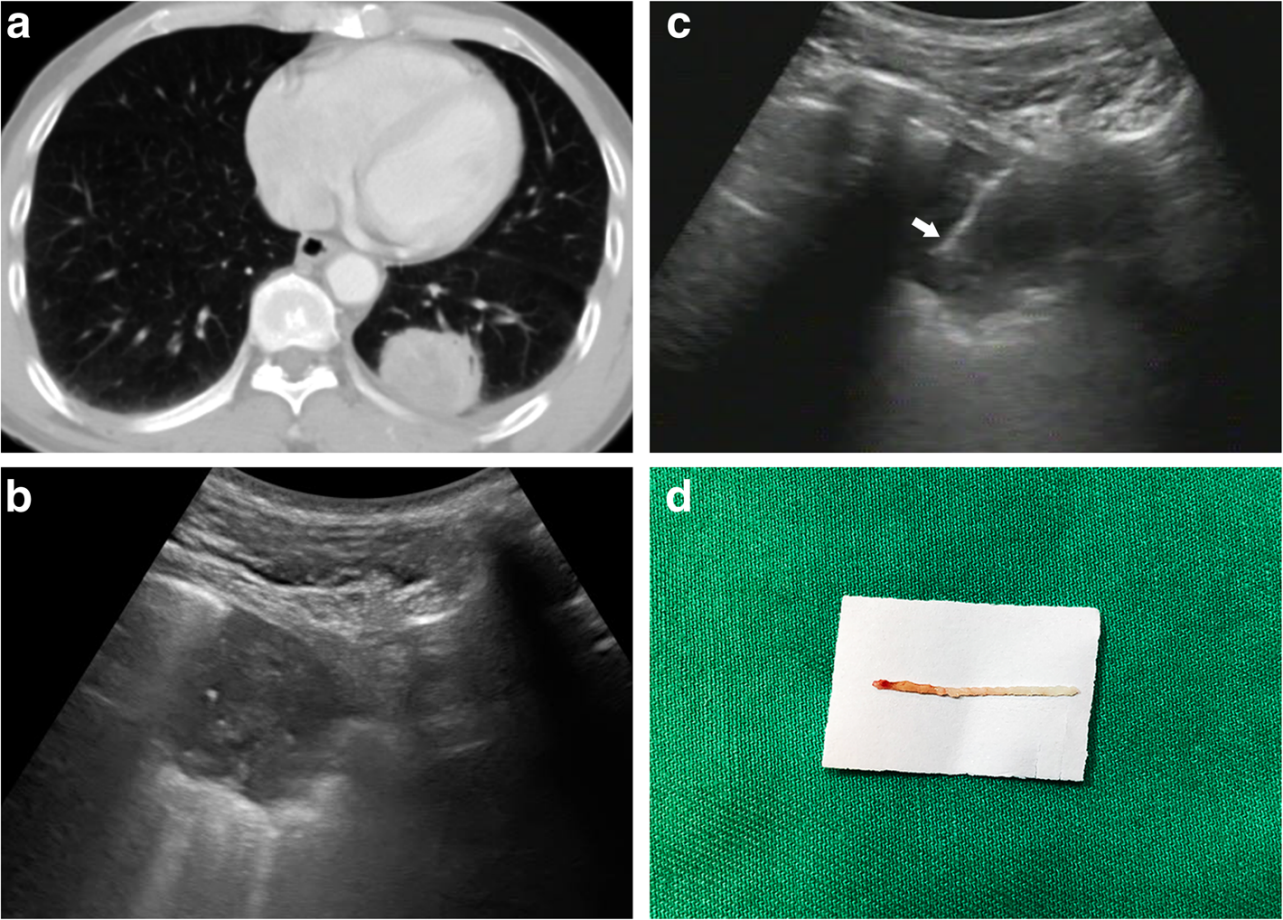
A 57-year-old patient with a peripheral lung lesion. A percutaneous core biopsy was requested to determine the nature of the lesion. a Axial contrast-enhanced computed tomography (CT) image revealing a round lung lesion in the left lower lobe with pleural contact. b Transverse gray-scale ultrasound (US) image obtained before biopsy revealing a hypoechoic lesion relative to the surrounding aerated lung. Positioning a transducer in the adjacent rib space shows broad pleural contact, providing a sonographic window for sampling. c Transverse gray-scale US image revealing a 16-gauge needle throw for core biopsy within the lesion (arrow), performed within a single breath-hold. d Color photograph of the biopsy sample
